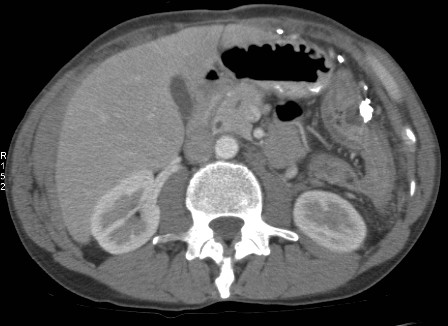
Axial CT image shows the abnormally thickened splenic flexure with surrounding hazy stranding in keeping with inflammation. The colobronchial fistula arises from this loop.
